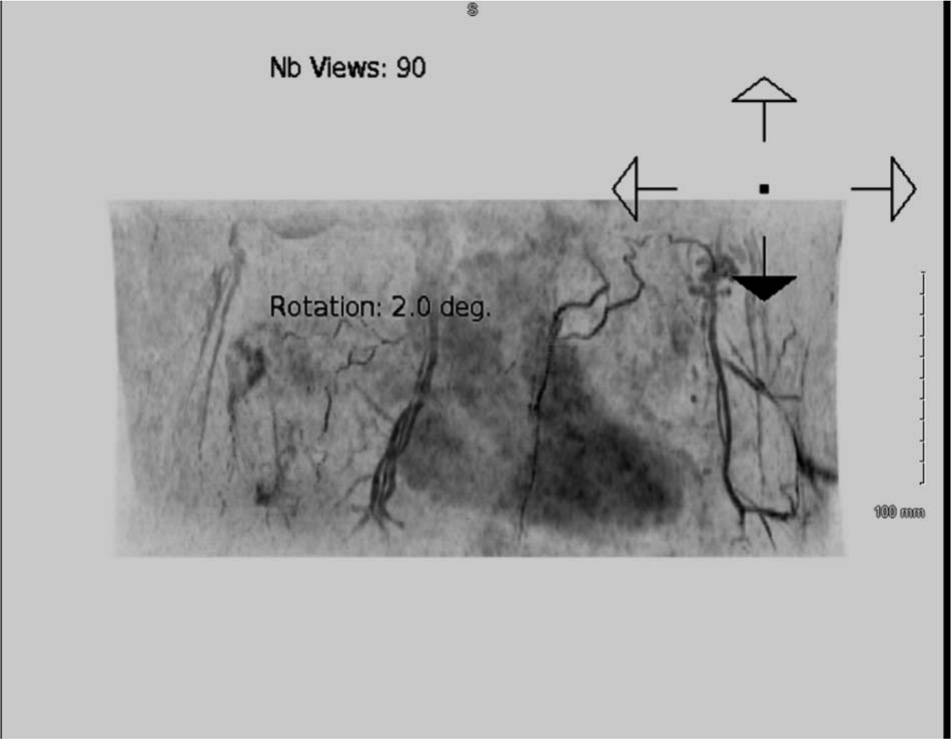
Pre-operative computed tomography (CT) angiography demonstrated robust vasculature perfusing the superior and lateral margins of the right breast, suggesting the planned infero-medial access incision would not de-vascularize the remaining breast tissue.
